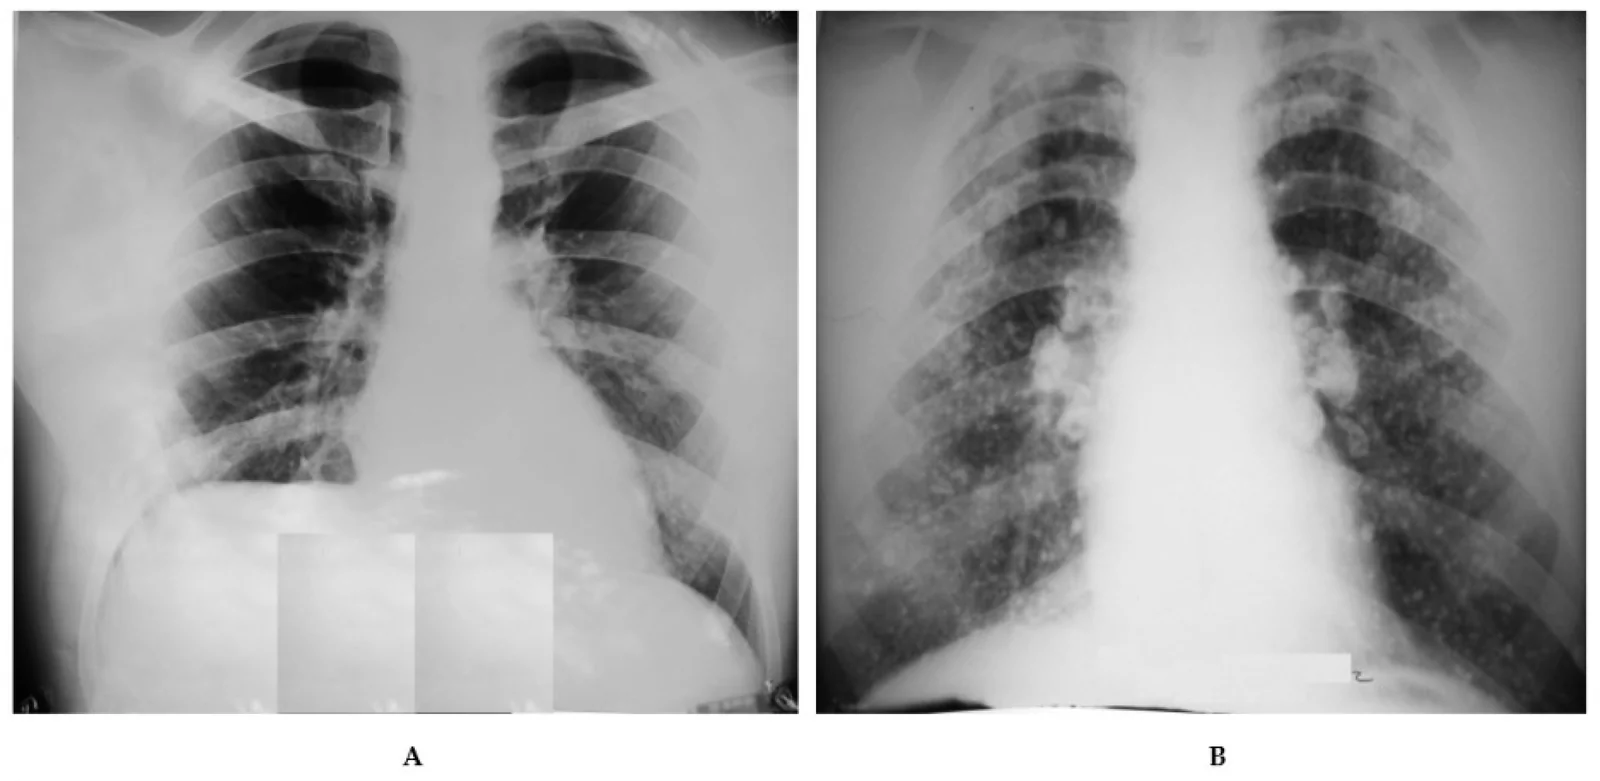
Chest X-rays: (A) normal; (B) silicosis with diffuse nodular and interstitial fibrous changes in the lung tissue.
